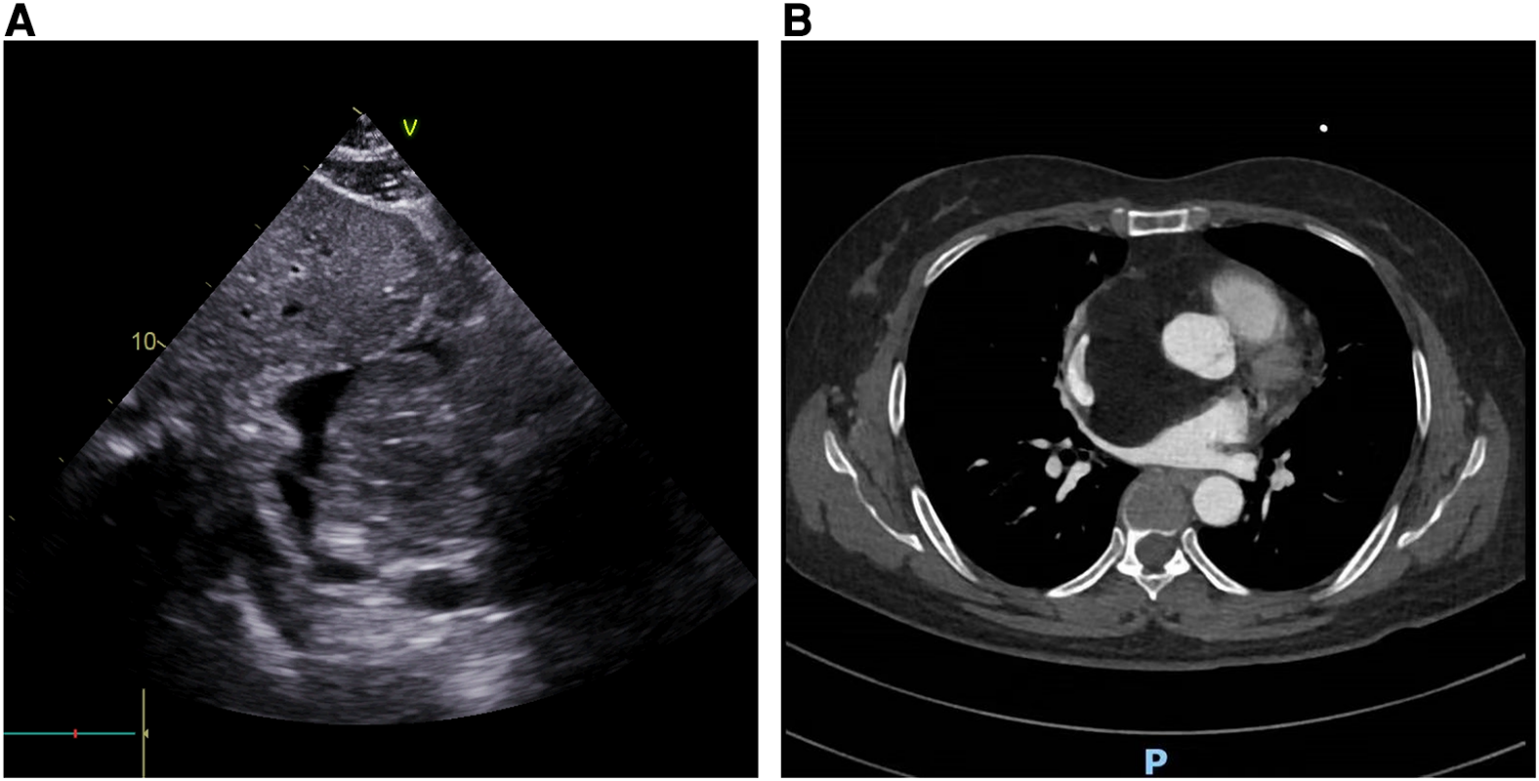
The imaging feature of the cardiac mass. (A) Echocardiography revealed a large mass attached to the interatrial septum; (B) CT revealed a low-density mass within the atrium, which compressed the superior vena cava and ascending aorta.
